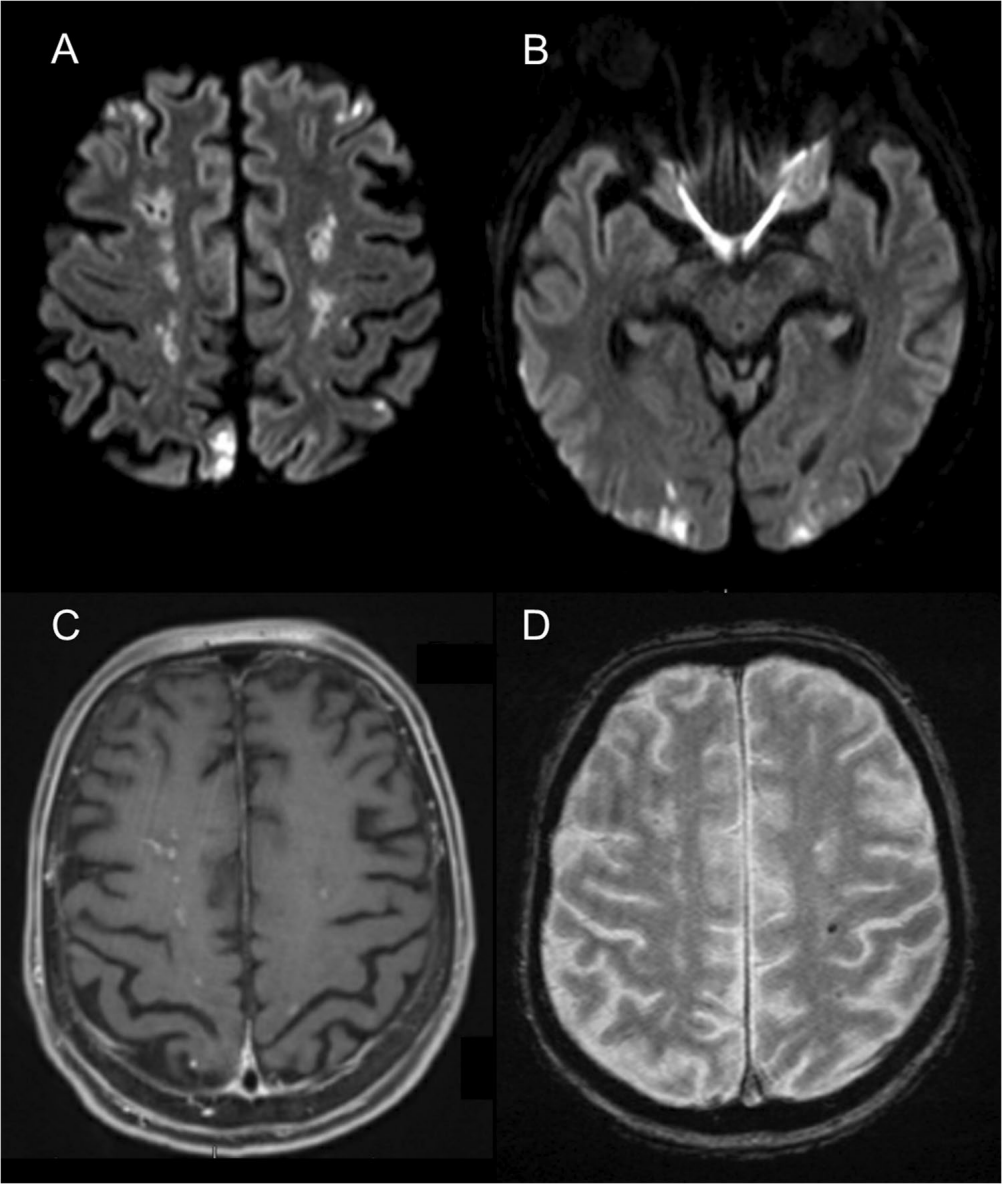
Brain magnetic resonance imaging showing multiple acute and subacute border zone infarcts in diffusion weighted imaging (A, B), some of which present gadolinium enhancement in T1 (C), and an example of a cerebral microbleed in T2-gradient echo (D)
